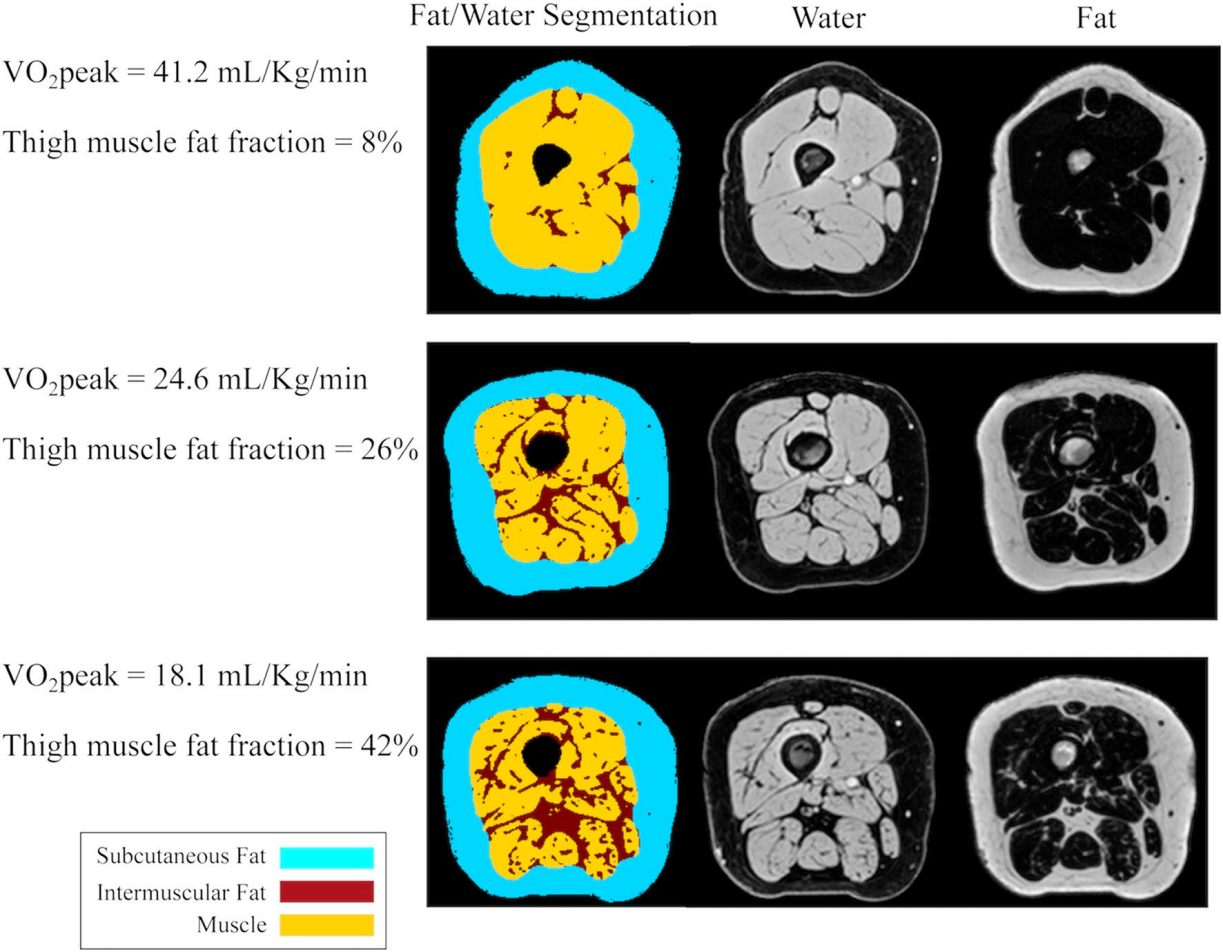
Representative participants with low, medium, and high thigh fat fraction with their respective water and fat suppressed images used to delineate the fat and muscle components. Axial right thigh images were acquired by magnetic resonance imaging and 40 cm of volume were analyzed starting 110 mm from the distal point of the femur to quantify absolute volumes of muscle and intermuscular fat (IMF). The thigh fat fraction was then calculated as: IMF/(IMF + muscle)*100%. Thigh fat fraction was inversely associated with VO_2_peak in both breast cancer survivors and matched controls.
